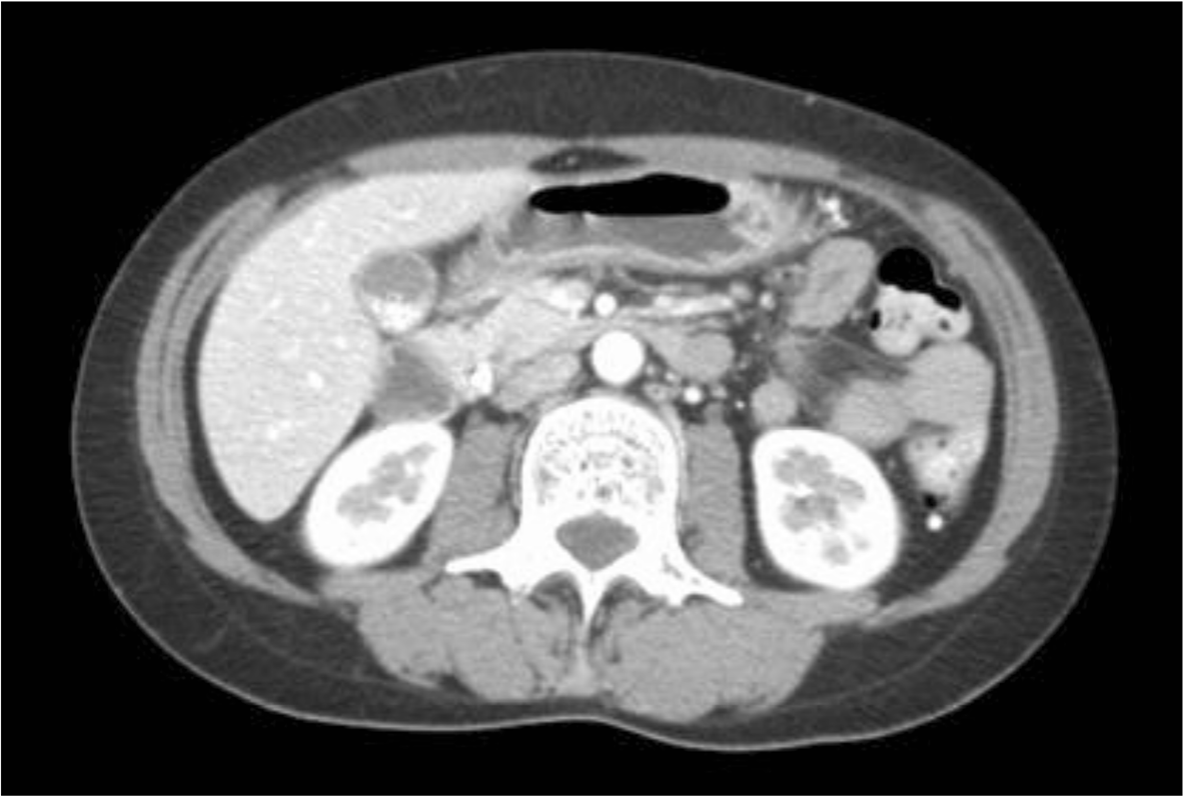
Abdominal computed tomography shows gallstones with gallbladder wall thickening
